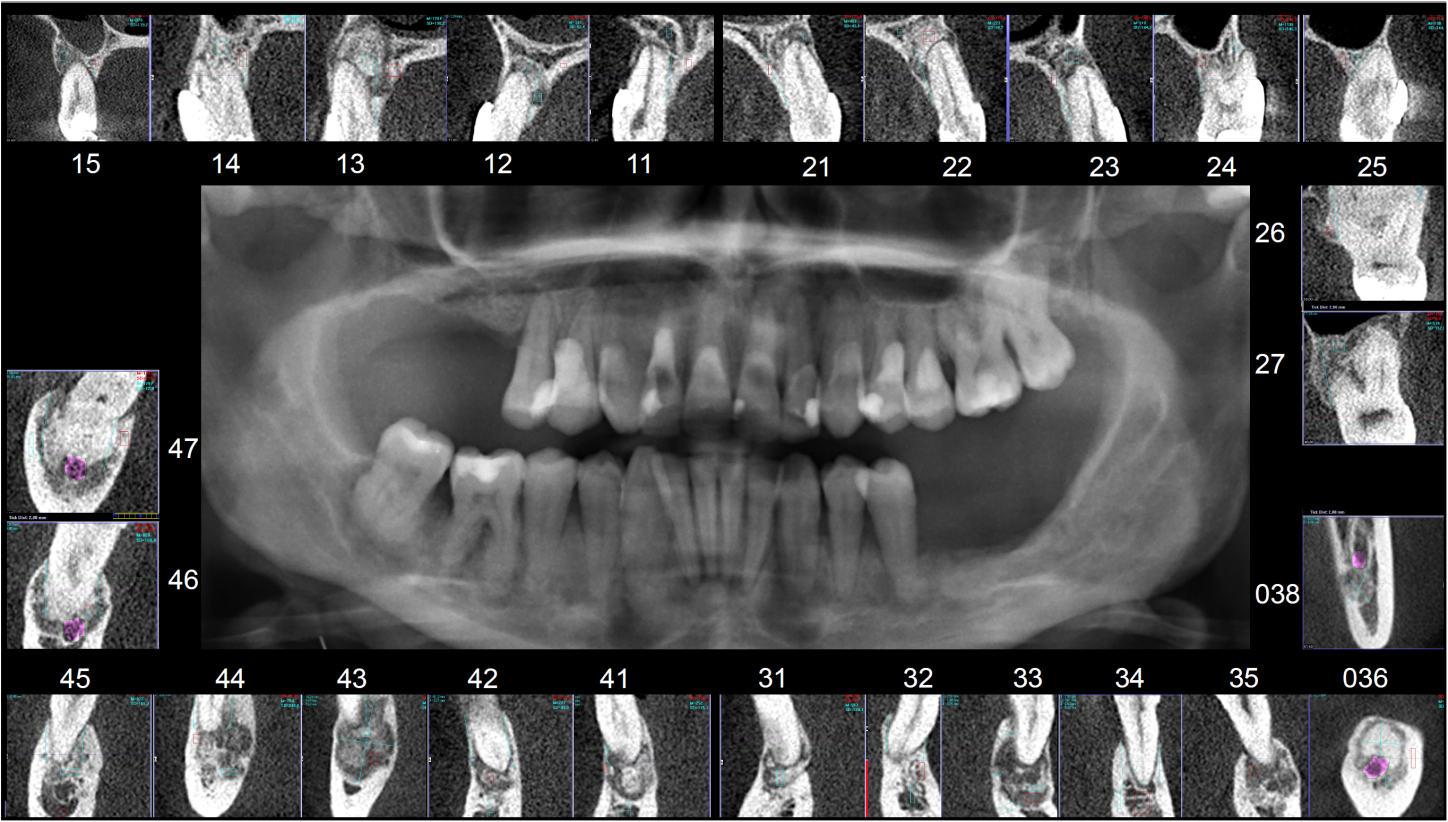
Center: CB‐CT‐ Orthopantomography‐Mode; Frame: Osteodensitometric detection by cross‐sectional images in the sagittal dimension from CB‐CT for each periapical region (CB‐CT‐O)
